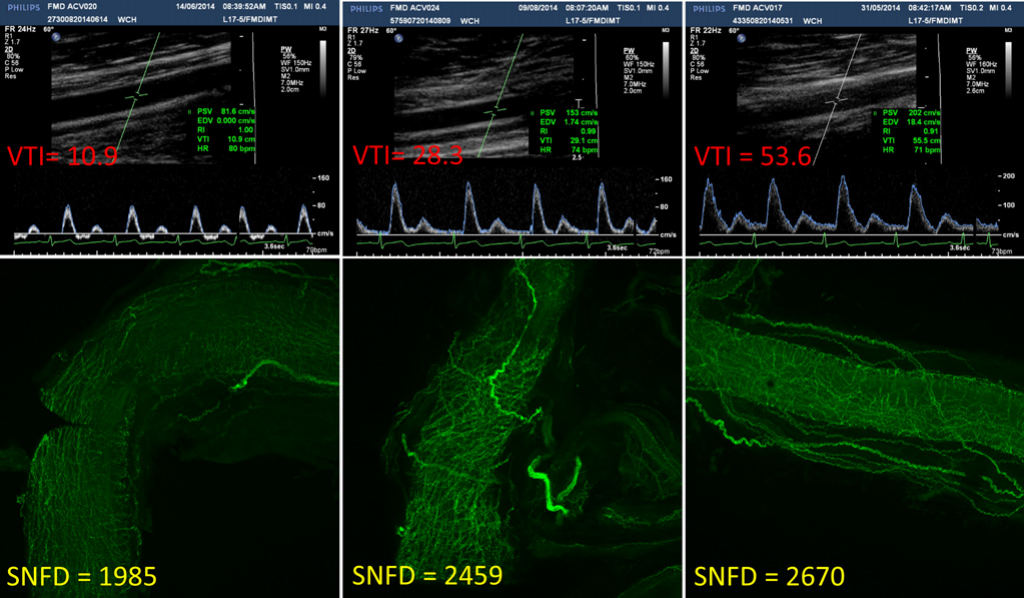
Blood flow velocity Doppler image (top panel), and the corresponding dorsal lingual arteries sympathetic nerve fiber images (bottom panel) for 3 representative participants. SNFD indicates Sympathetic Nerve Fiber Density; VTI, velocity time integral.
